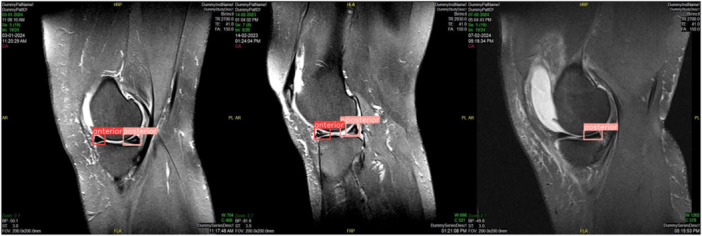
True positive (TP), false positive (FP) and false negative (FN) results in the sagittal view of magnetic resonance imaging scans for meniscus tear detection using the YOLOv8 and EfficientNetV2 model.
